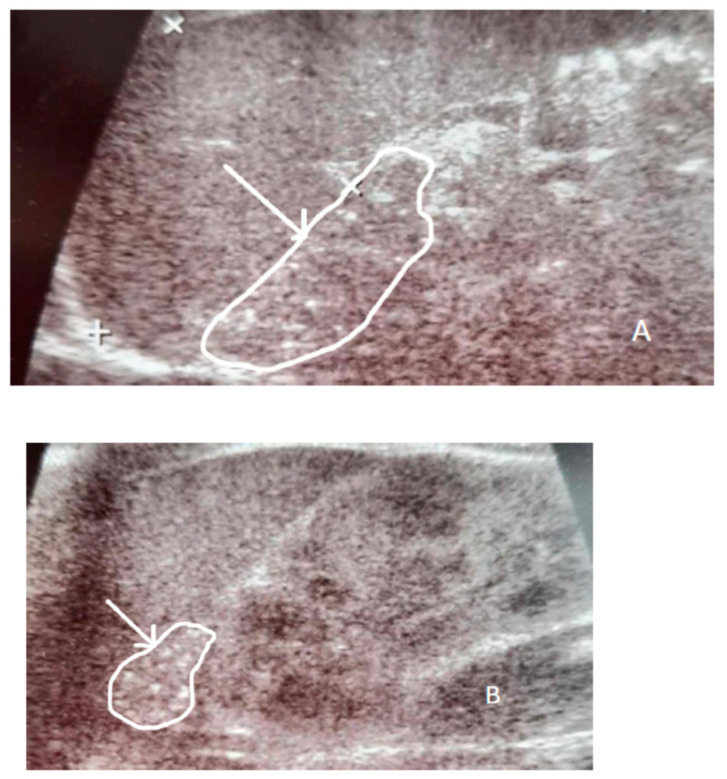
Ultrasound scans showing calcifications in (A)—right adrenal gland and (B)—left adrenal gland (white arrows, white line encircles the adrenal glands).
